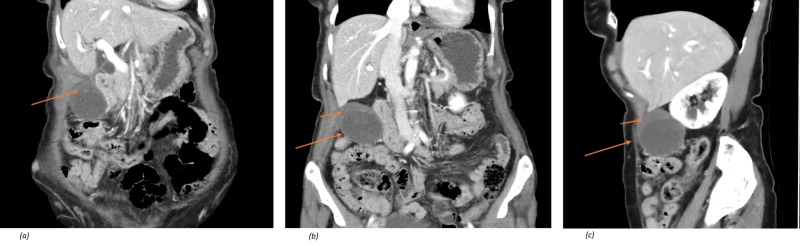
Computed tomography (CT) of torted gallbladder. (a) V-shape superior portion of gallbladder with asymmetrical thickening of gallbladder wall. (b) Low and horizontal lying of gallbladder. (c) Gallbladder lower than inferior liver edge, rather than typical anatomical position within gallbladder fossa. Short arrows - Hypoattenuation of the gallbladder wall compared with surrounding visceral structures.
